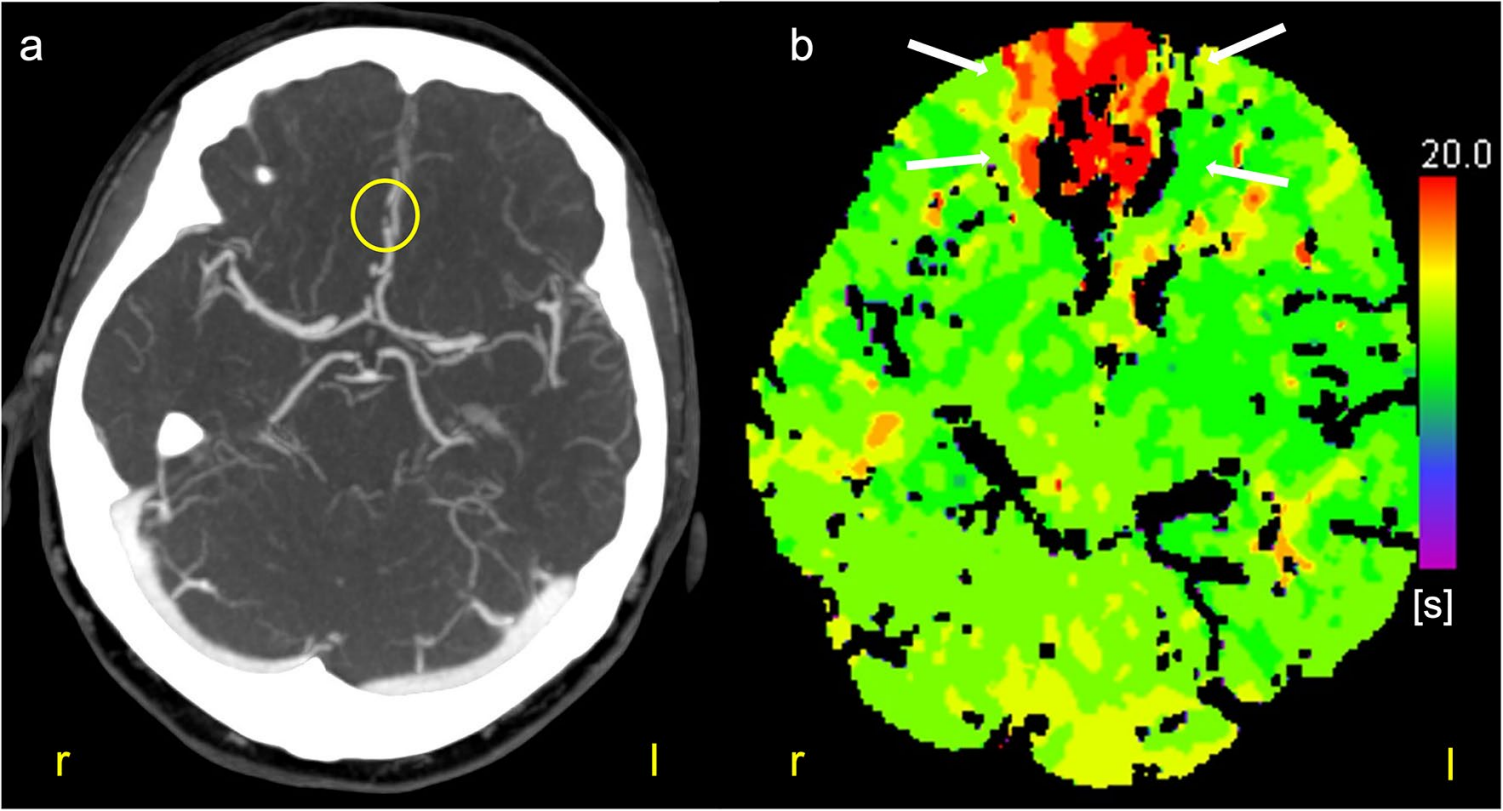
Various computed tomographic (CT) images of a 67-year-old female patient with reversible cerebral vasoconstriction syndrome (RCVS). CT-angiography after acute left leg-stressed hemiparesis (a). At the transition of A2-to-A3-segment of the right anterior cerebral artery (ACA), a short-stretch vessel stenosis is found, compatible with a vasospasm (yellow circle). In the anterior circulation (right ACA territory) (b), there is a frontal time-to-peak-delay (TTP) in the perfusion-CT (white arrows, s: second)
